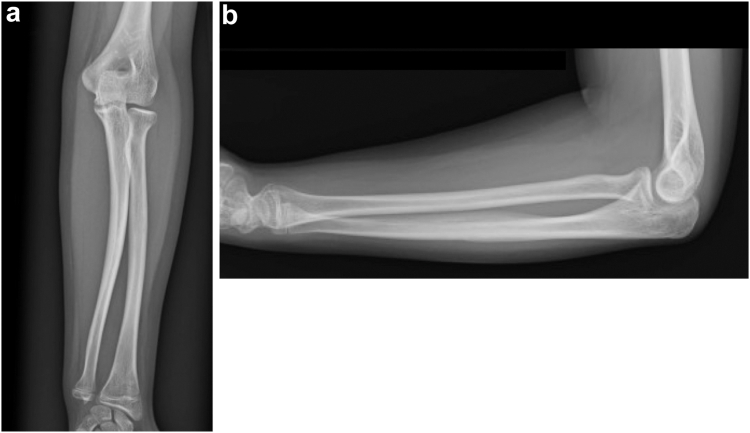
Three months postinjury. Radiographs showed complete healing of the radius and ulna fractures, with the radial head maintaining normal alignment. (a) Antero–posterior view. (b) Lateral view.
